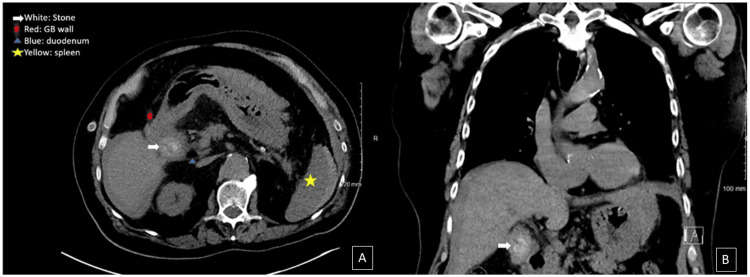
CT thorax on day one of hospitalization A: Transverse section shows a mass (white arrow) that is notably present within the gallbladder (red dot); B: Coronal section showing the mass (white arrow) notably present within the gallbladder
